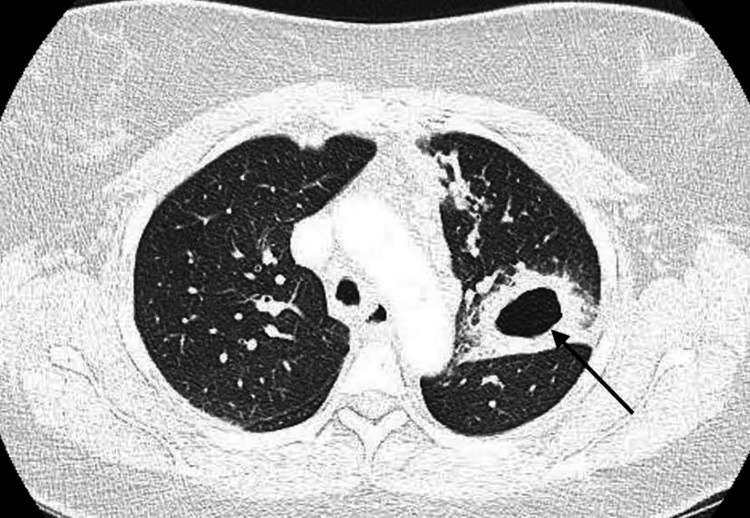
Computed tomography angiography showing the left upper lobe thick-walled cavity (arrow) 4.8 x 3.4 cm in diameter
